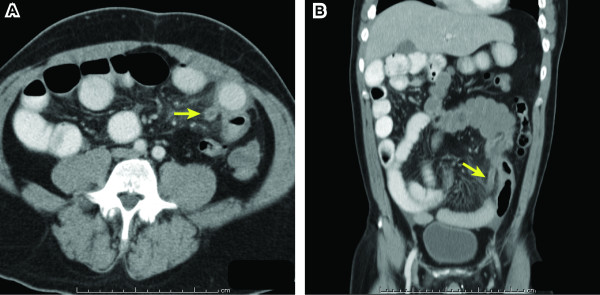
Abdominal CT: Atypical acute appendicitis. Axial spiral CT with oral and IV contrast (A) and coronal multiplanar reconstruction (B) demonstrate dilated appendix in the left mid-abdomen (arrows) with adjacent fat stranding.
